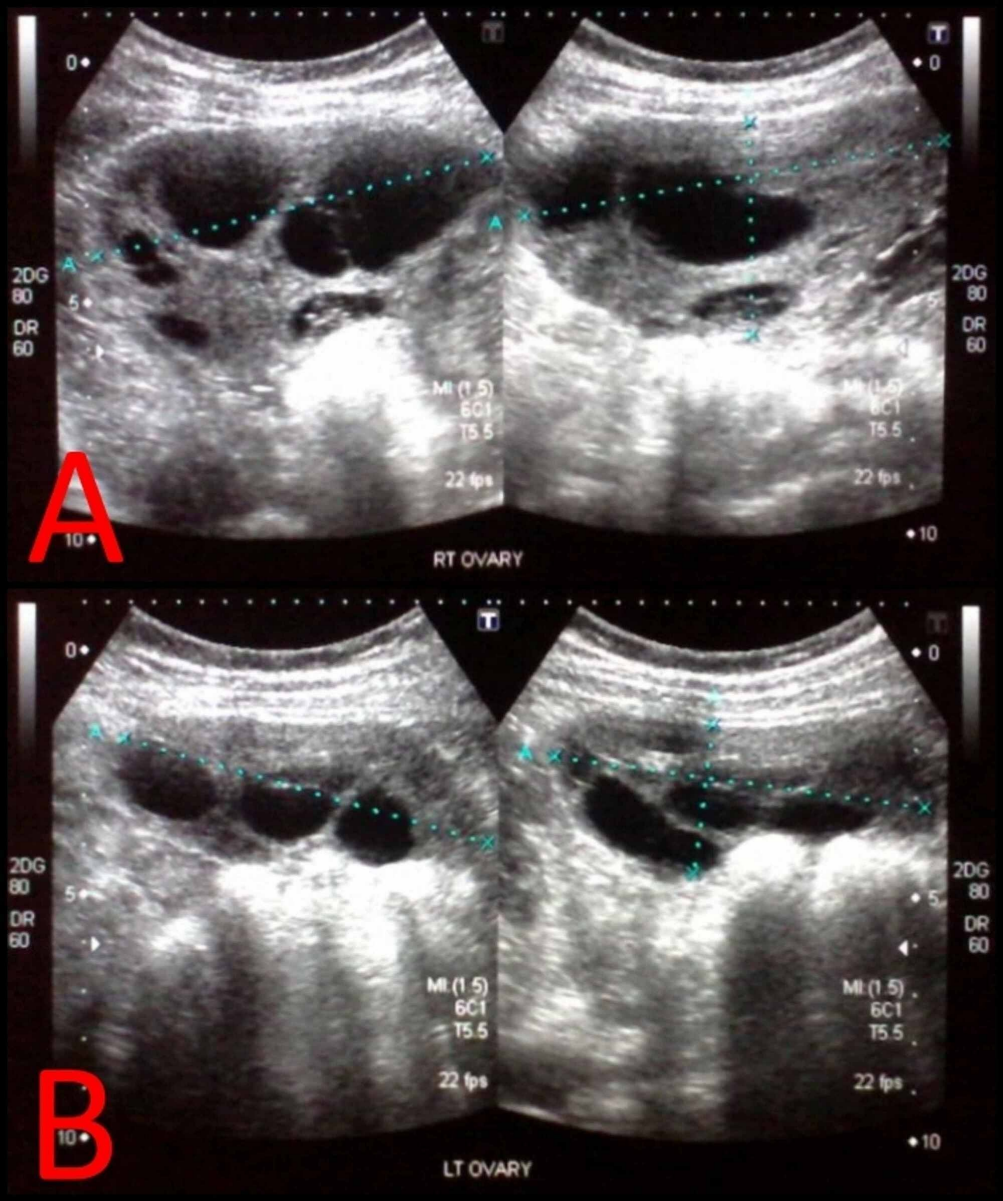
Post-operative ultrasound showing reduction in the size of (A) right and (B) left ovaries
